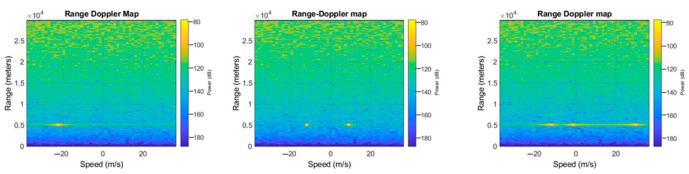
Example of range-Doppler maps generated with fixed positions at different speeds of (respectively, from the left to right) 1, 2, 3 targets.
